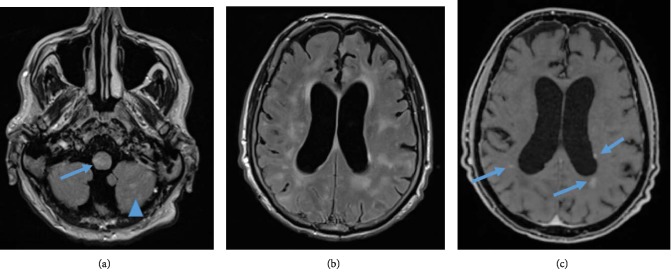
MRI brain 4 months after presentation. FLAIR transversal images (a and b) and post contrast image (c) demonstrating marked brain atrophy and new abnormal hyperintensities in the spinal cord (arrow, a) and cerebellum (arrowhead, a) , throughout the periventricular and subcortical white matter (b) and new enhancement (arrows, c).
